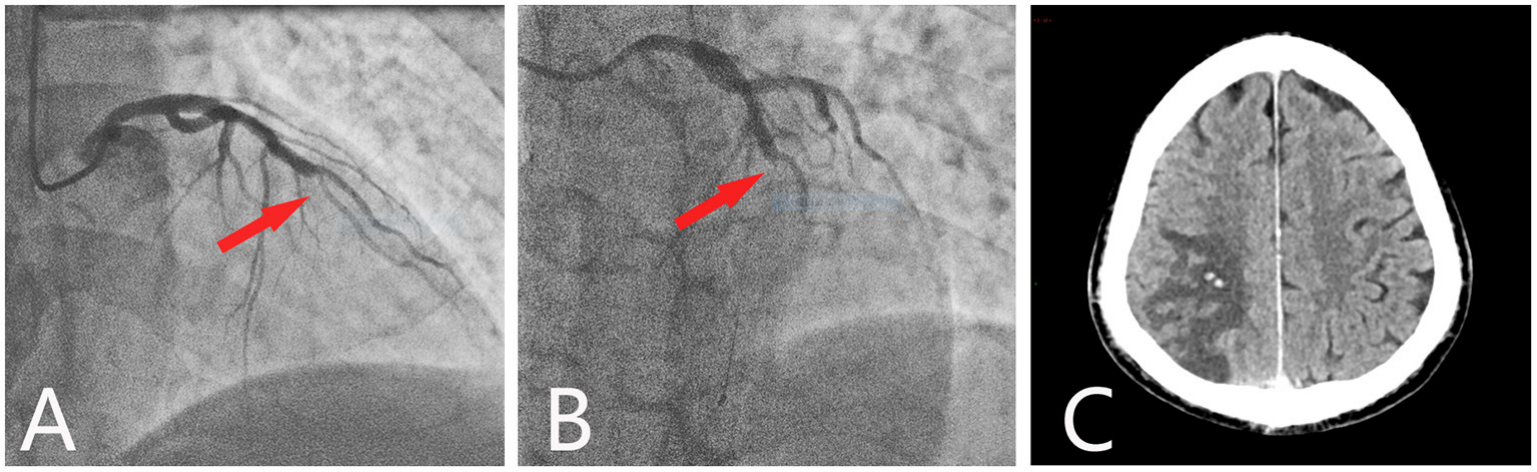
Coronary angiography and cerebral CT. Coronary angiography showed a total occlusion at the mid-left anterior descending branch (red arrow). (A) Cranial view. (B) Left cranial view. (C) Axial cerebral CT showed hypodensity in the right parietooccipital lobe, with two spotted hyperdense areas.
